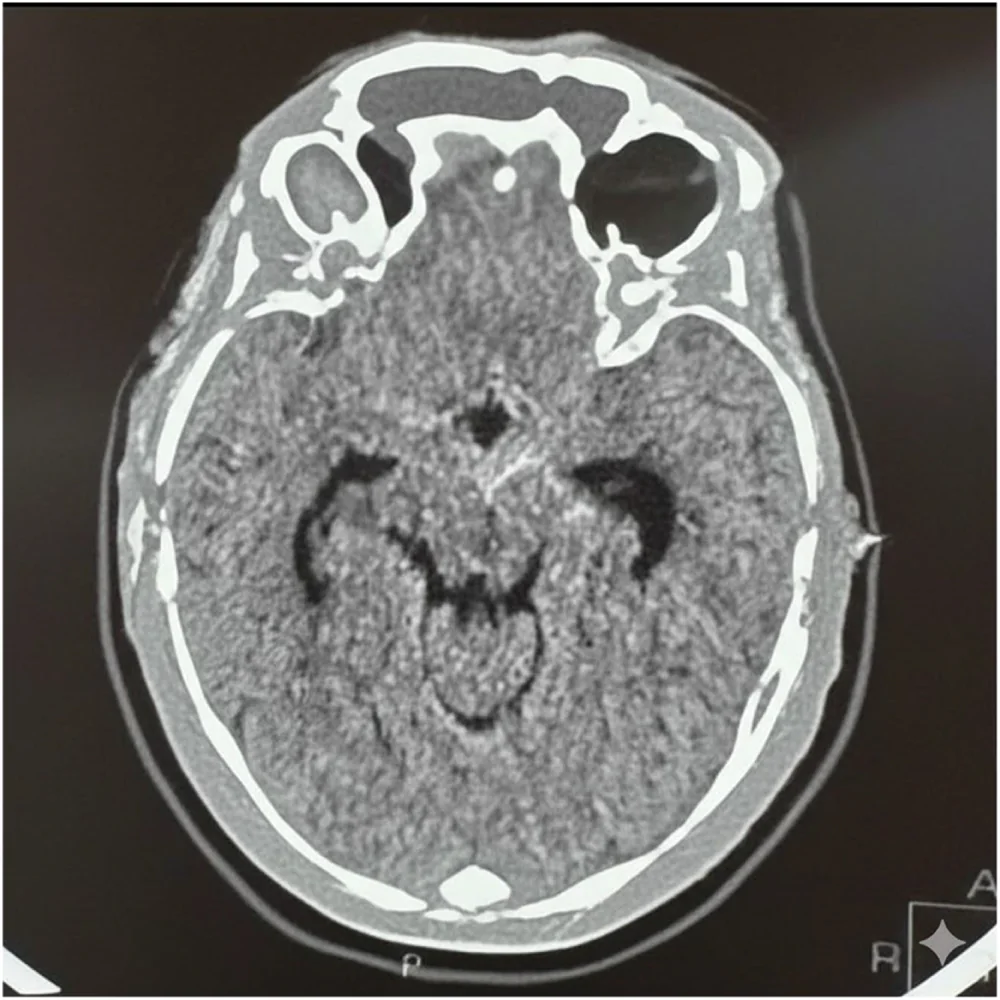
Non-contrast axial CT scan. The imaging shows normal brain parenchyma with preserved sulcal patterns and no acute hemorrhagic or obstructive features. In cases of cerebral venous thrombosis, a native CT is often normal, serving primarily to exclude other acute causes of headache.
